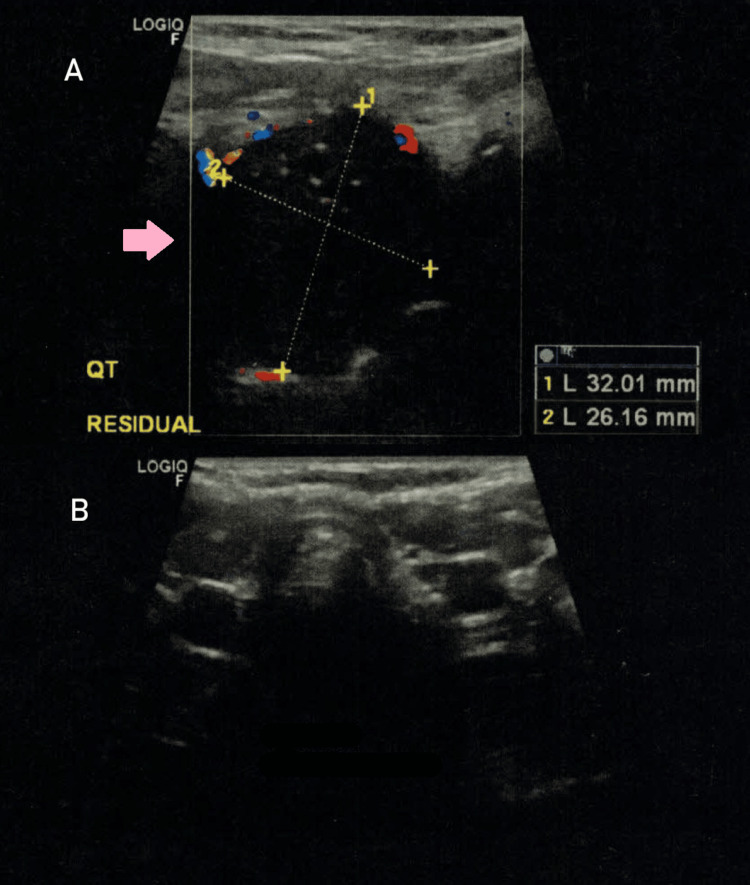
Anterior neck ultrasound (transverse view). (A) Cystic mass of 32x26 mm in the infrahyoid region (pink arrow), with regular edges, dense content and small peripheral calcifications, interpreted as a residual cyst (B) Small (atrophic) thyroid gland with heterogeneous parenchyma
